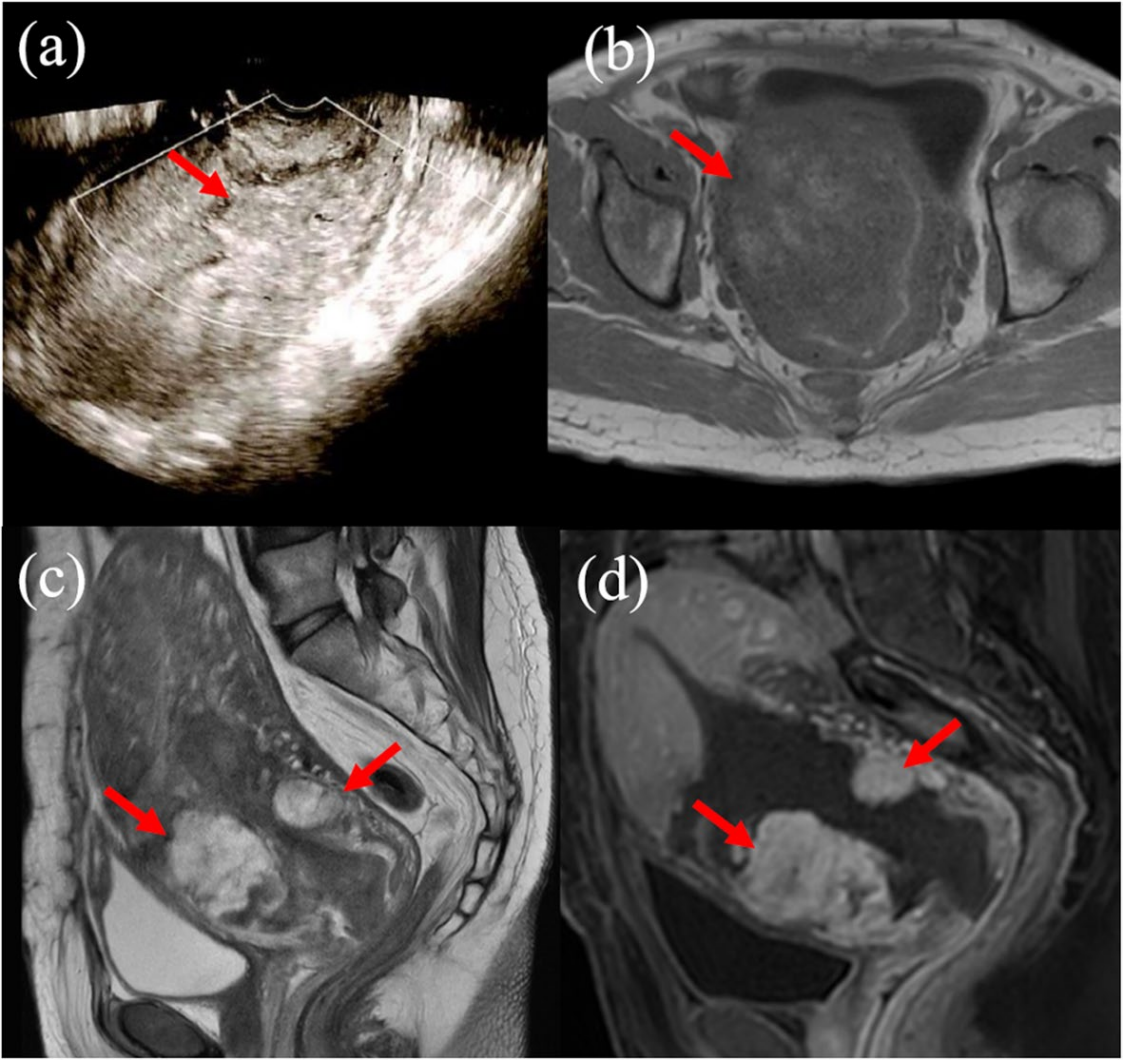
These images were diagnosed for retained products of conception (RPOC) in pregnant patients with placenta previa after operation. RPOC was represented as an echogenic mass at transvaginal color Doppler ultrasonography (US) after one day of operation (a), which was detected no flow, a heterogenous signal area at T1-weighted magnetic resonance imaging (MRI) (b), and a high signal area at T2-weighted MRI (c) and contrase-enhanced T1-weighted MRI (d) (red arrow heads show)
